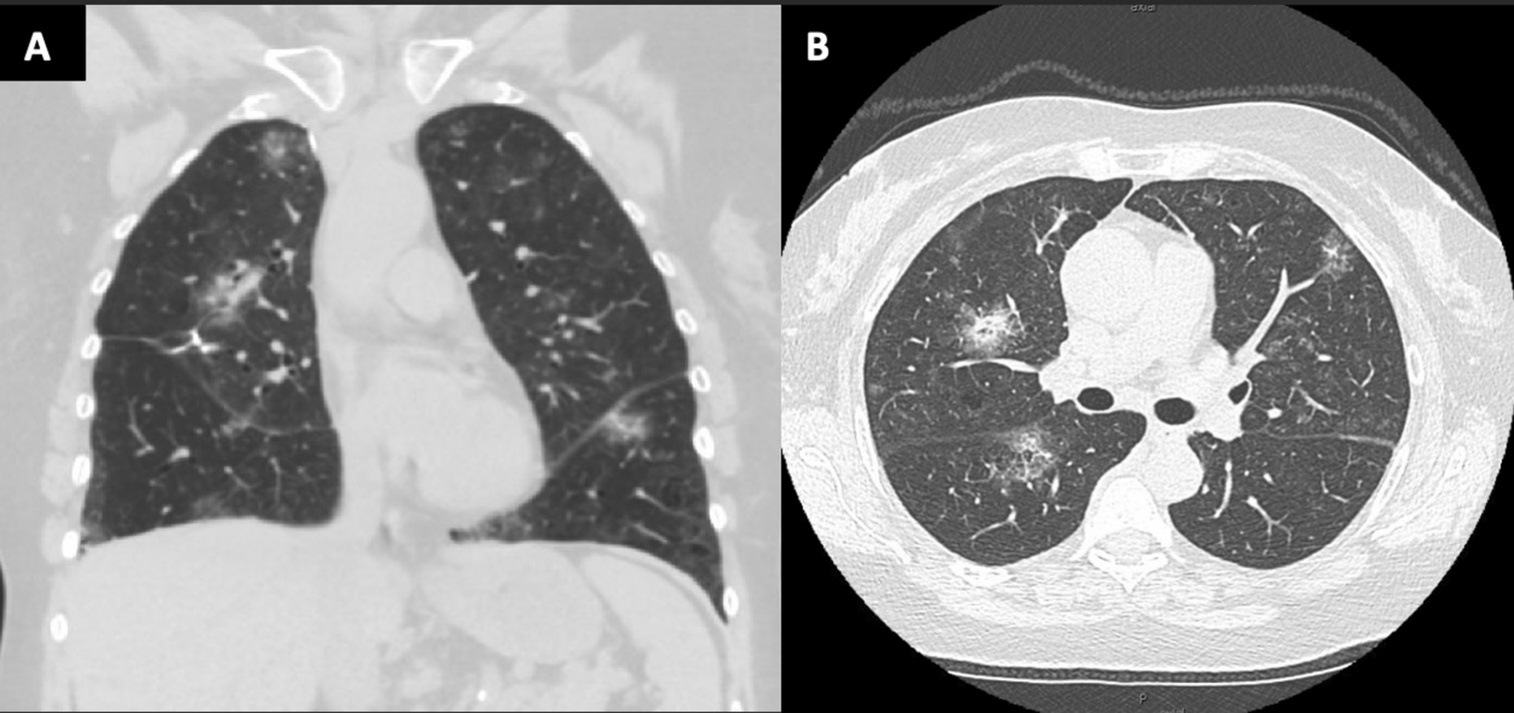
(A) Coronal view computed tomography (CT) of the chest shows waxing and waning nodules with an 8 mm semisolid right middle lobe (RML) lesion and increased bilateral ground glass opacities one year after rituximab treatment and two years after the initial diagnosis. (B) Axial view chest CT scan.
